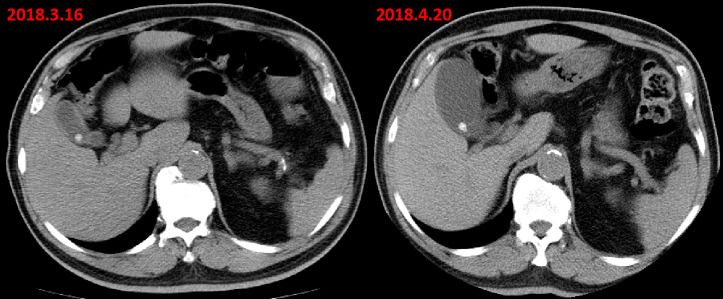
Axial abdominal CT scan (before colon surgery) revealed a slightly uniform
